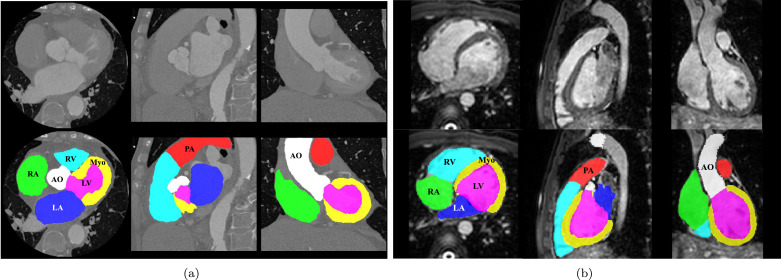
Examples of cardiac images and WHS results: (a) displays the three orthogonal views of a cardiac CT image and its corresponding WHS result, (b) shows example cardiac MRI data and the WHS result. LV: left ventricle; RV: right ventricle; LA: left atrium; RA: right atrium; Myo: myocardium of LV; AO: ascending aorta; PA: pulmonary artery.
